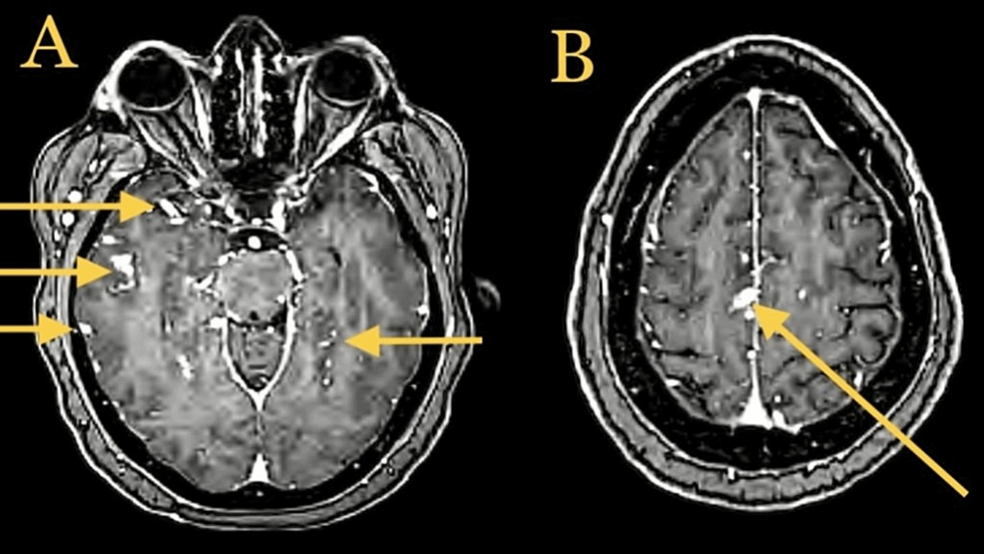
MRI head with contrast, axial T1 demonstrating abnormal diffuse enhancement (yellow arrows in image A), with the most significant area (4 mm × 7 mm) at the vertex (yellow arrow in image B)
